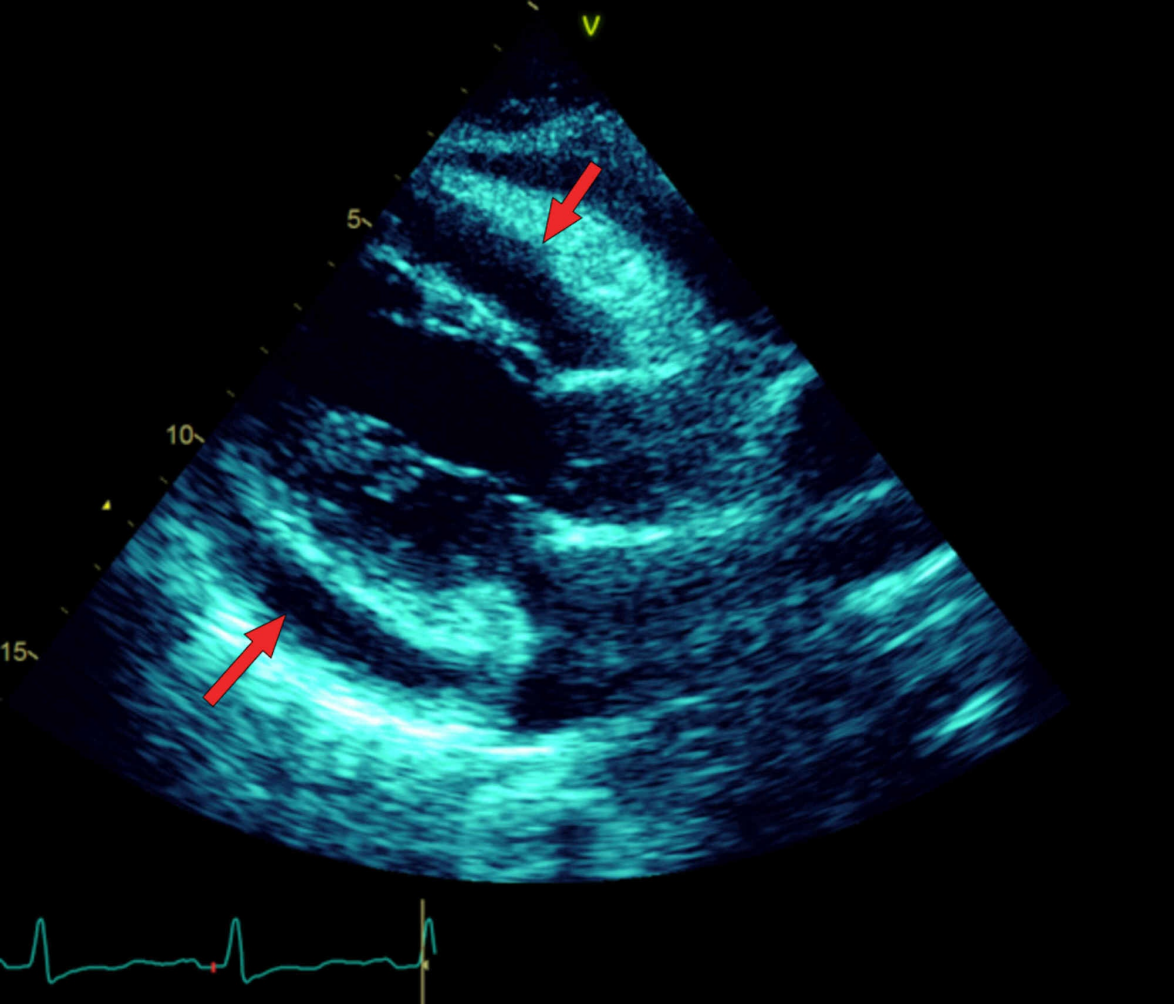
Red arrows: circumferential pericardial effusion (echocardiogram).
